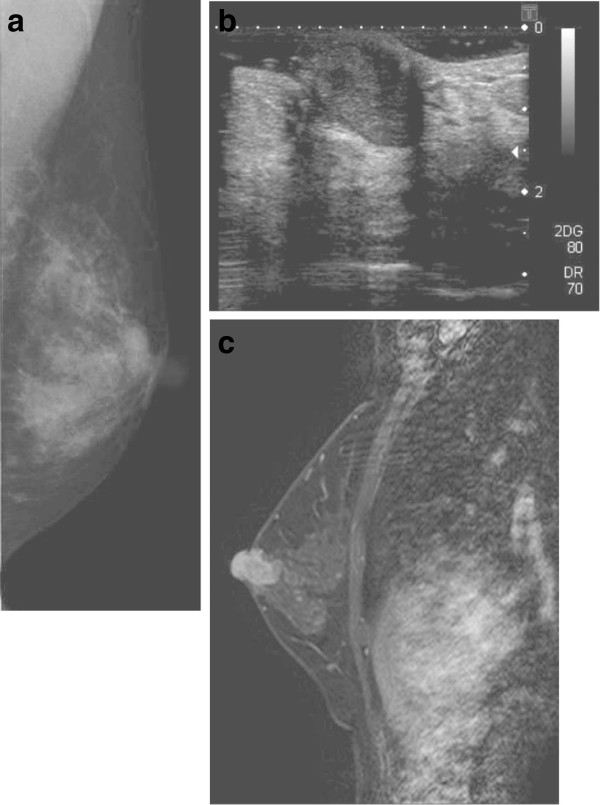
Mammography, sonography and MRI images. (a) Mammography revealed a well-defined, oval-shaped nodule with uniform density and no microcalcification. (b) Sonography and (c) MRI revealed that the mass under the nipple was continuous with the top of the nipple, showing adenoma of the nipple projecting out of the nipple. MRI, magnetic resonance imaging.
